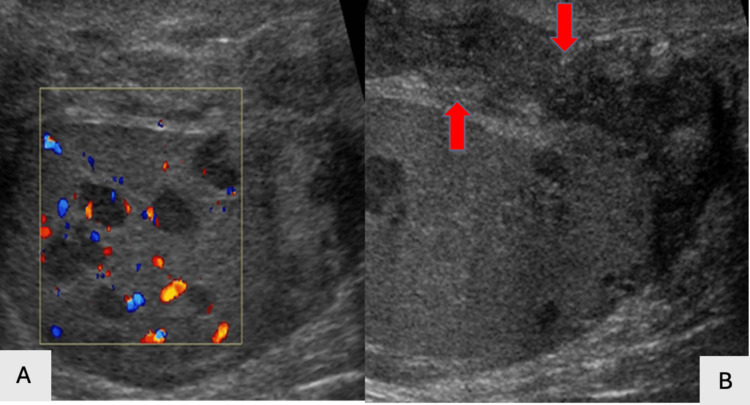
Color Doppler (23A) and grayscale (23B) images through the testicle demonstrate multiple microabscesses in a TB patient (yellow rectangle and red arrows).
